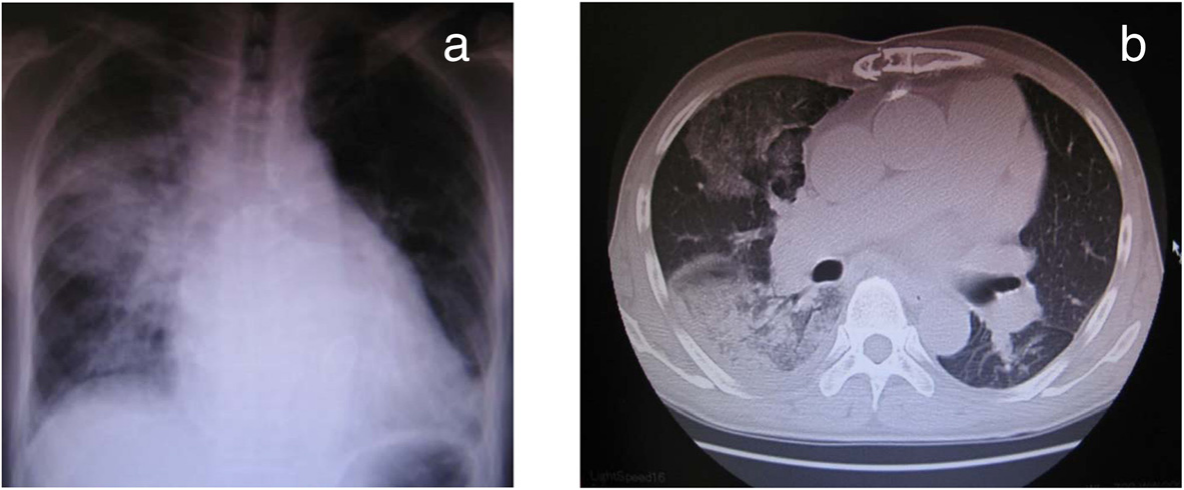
Chest radiograph (a) and chest computed tomography (b) at hospital admission
